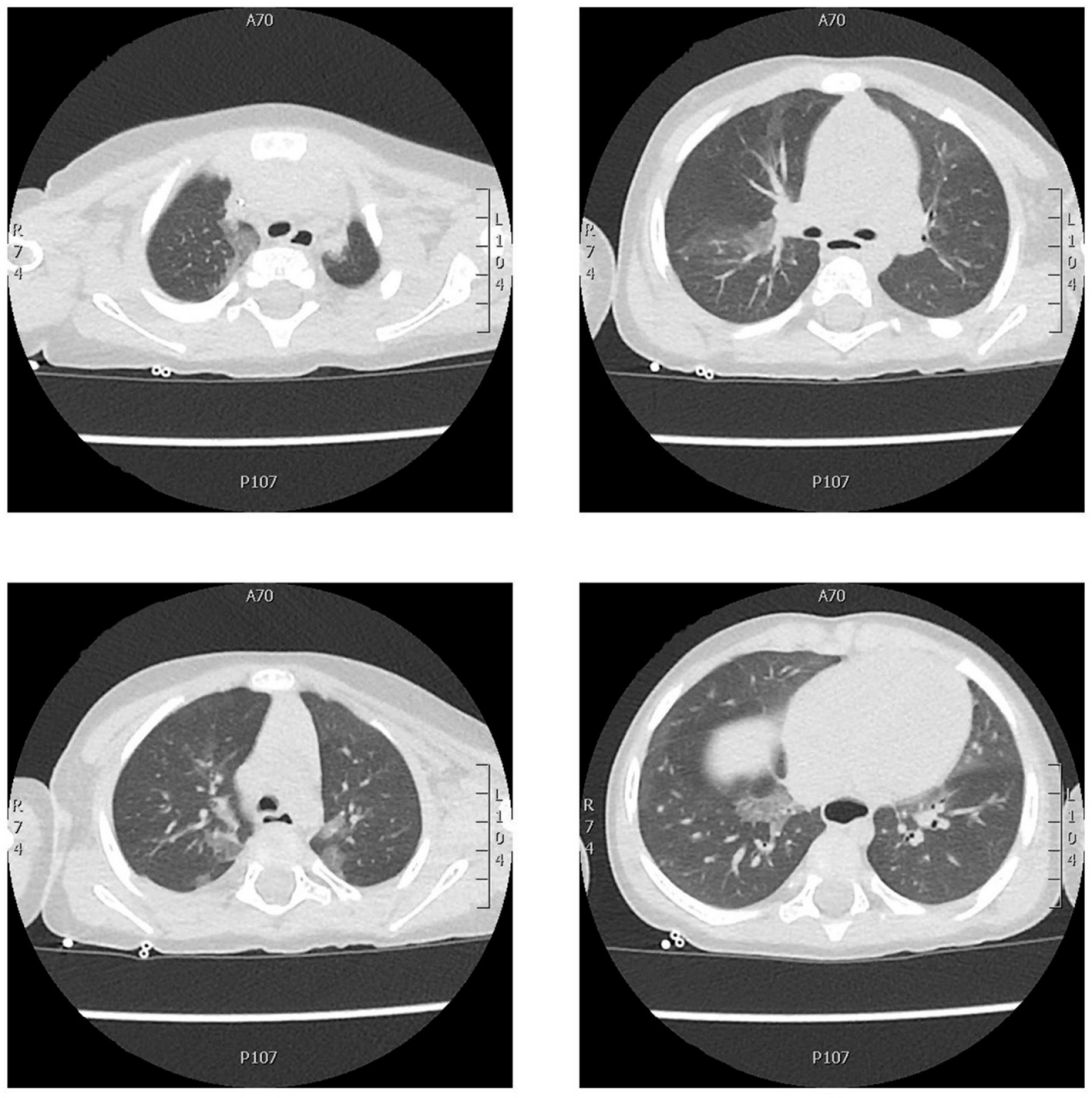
Chest CT axial scan showing areas of central and peripheral ground-glass opacity extending from the apex to the base of both lungs with no pleural effusion. The estimated total lung involvement was 5–10%.
